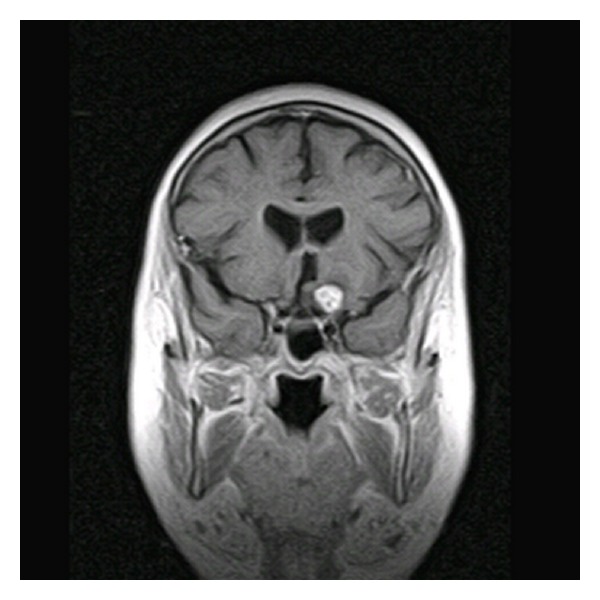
Axial MRI demonstrates avidly enhancing mass in the left paraclinoid region.
